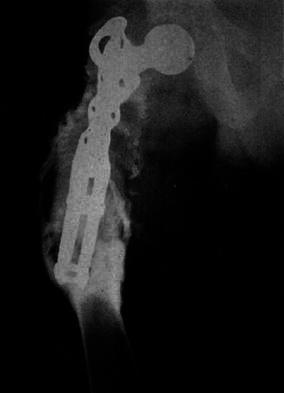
Plain radiograph of the original vitallium endoprosthesis from Moore and Bohlman [5]. Reprinted with permission from JBJS
